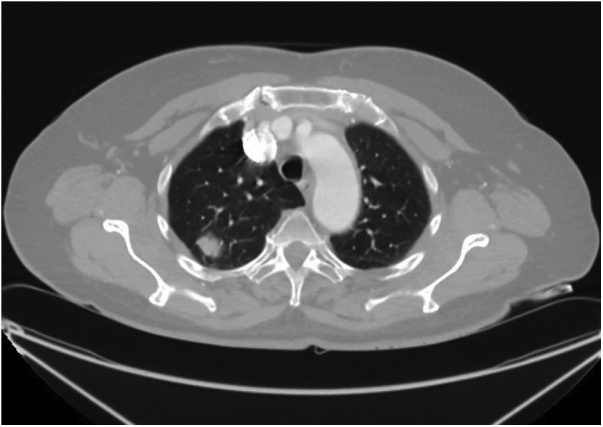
Chest CT image showing malignant lesion in the apical segment of the right upper lobe.
